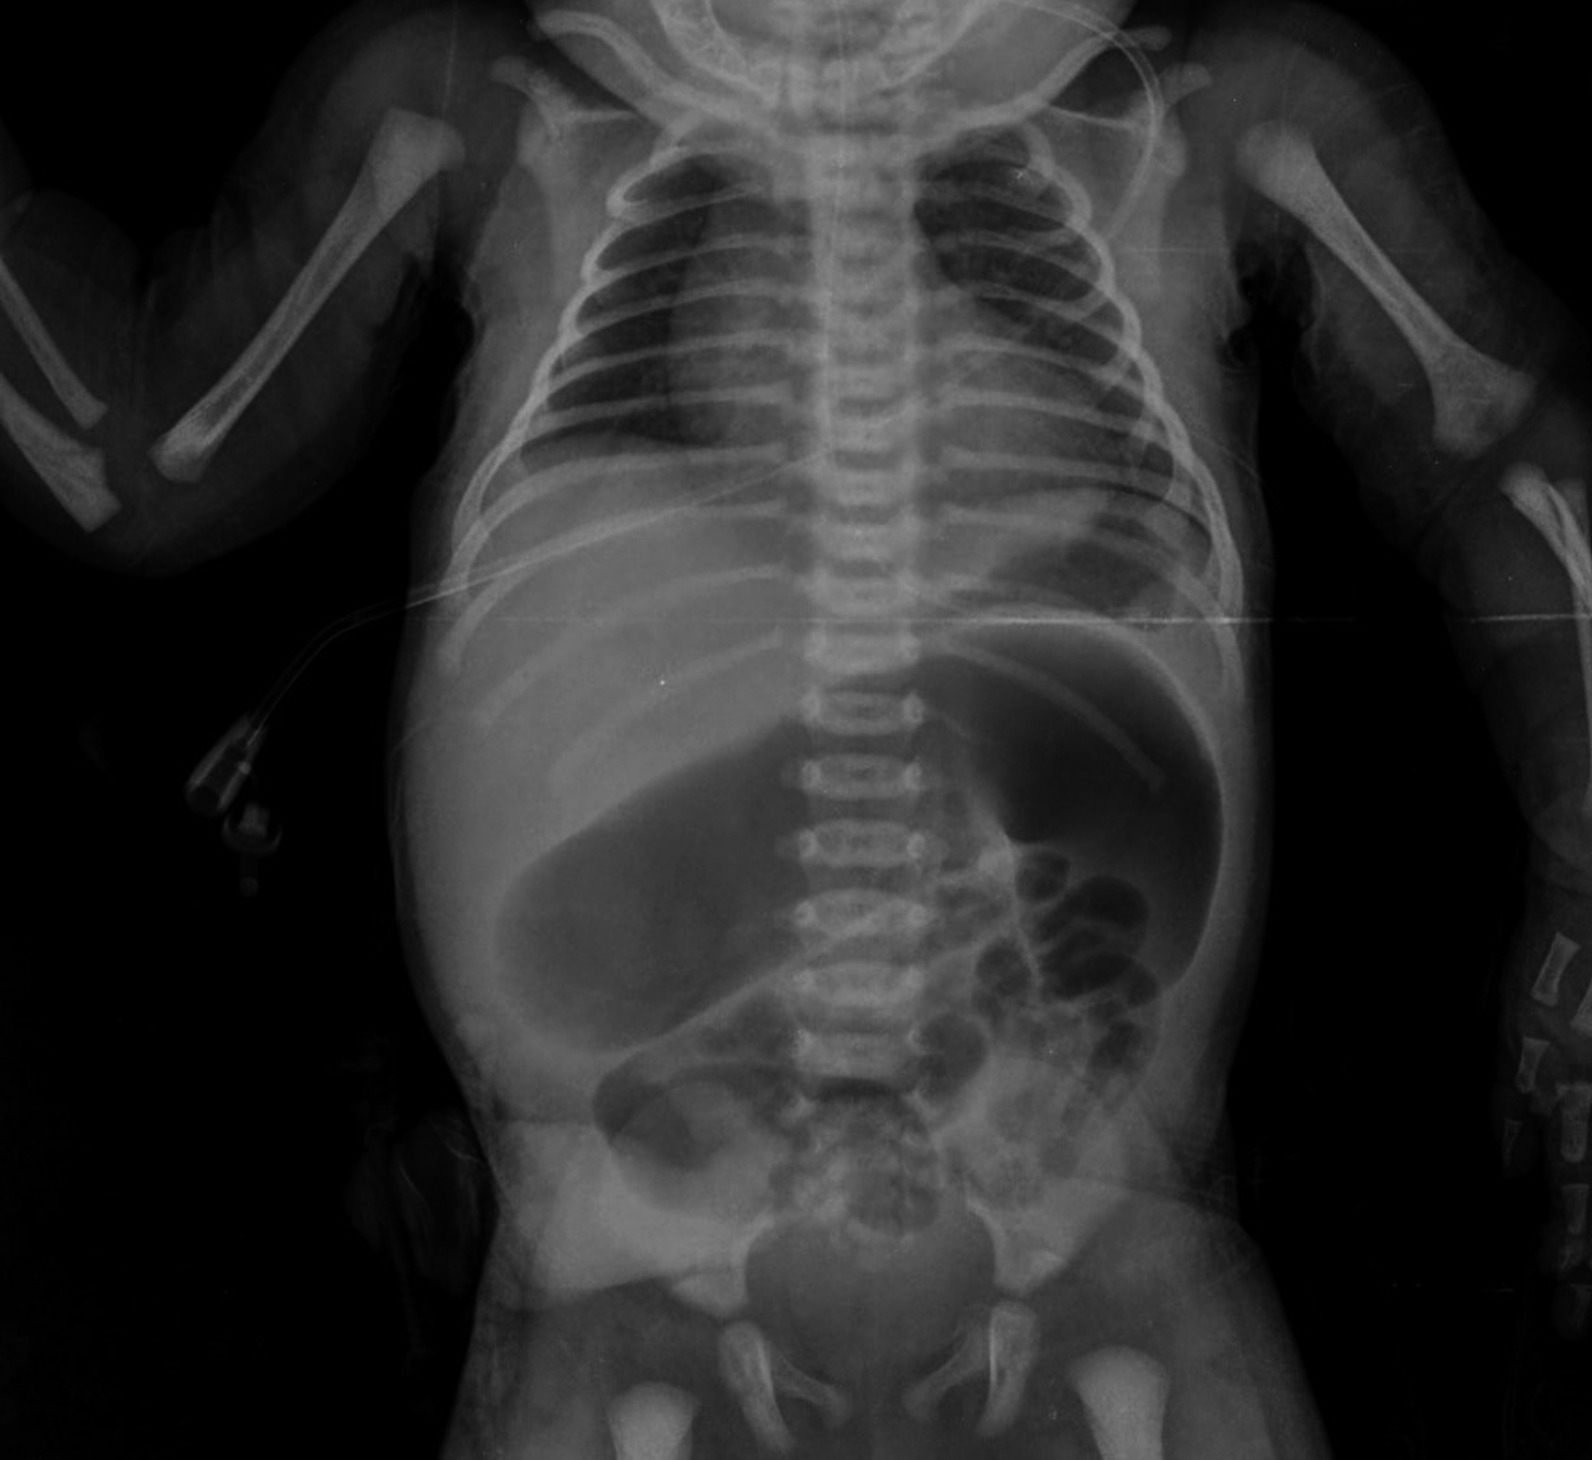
Plain radiograph of the patient shows gasless rectum and dilated intestines, evident of complete distal obstruction with multiple loops
